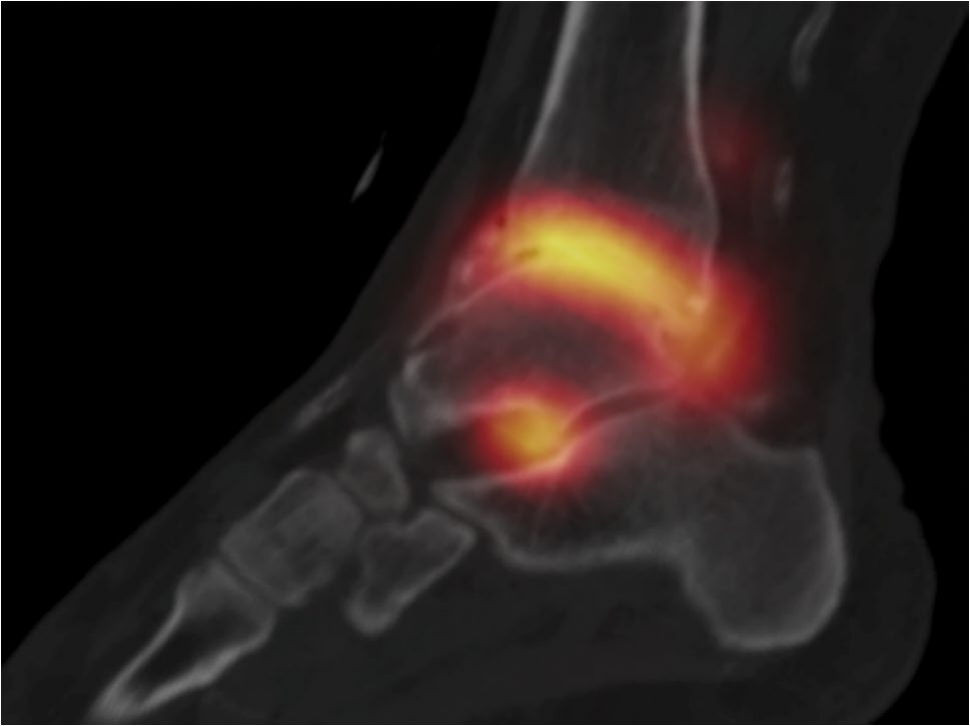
Distribution scan by SPECT/CT 30 min after intraarticular injection of 74 MBq [^186^Re]rhenium sulfide in an ankle joint of a patient with rheumatoid arthritis
